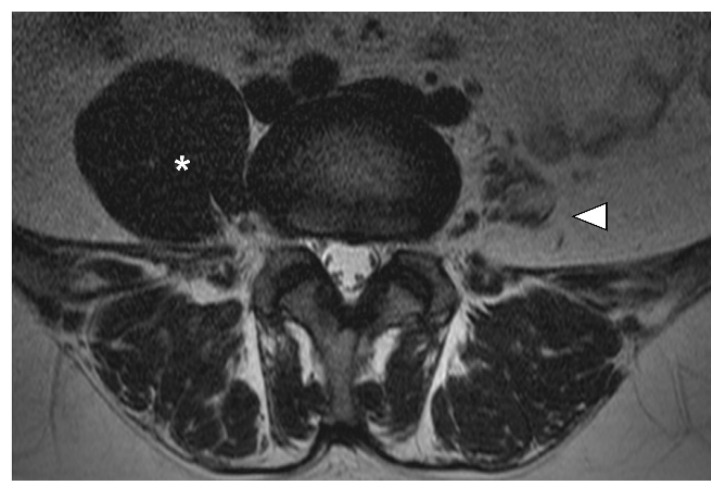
Magnetic resonance imaging (MRI) of the lumbar region. Left iliopsoas muscle atrophy (white arrowhead) compared to the right side (star point) observed on lumbar MRI at the time of the initial visit.
